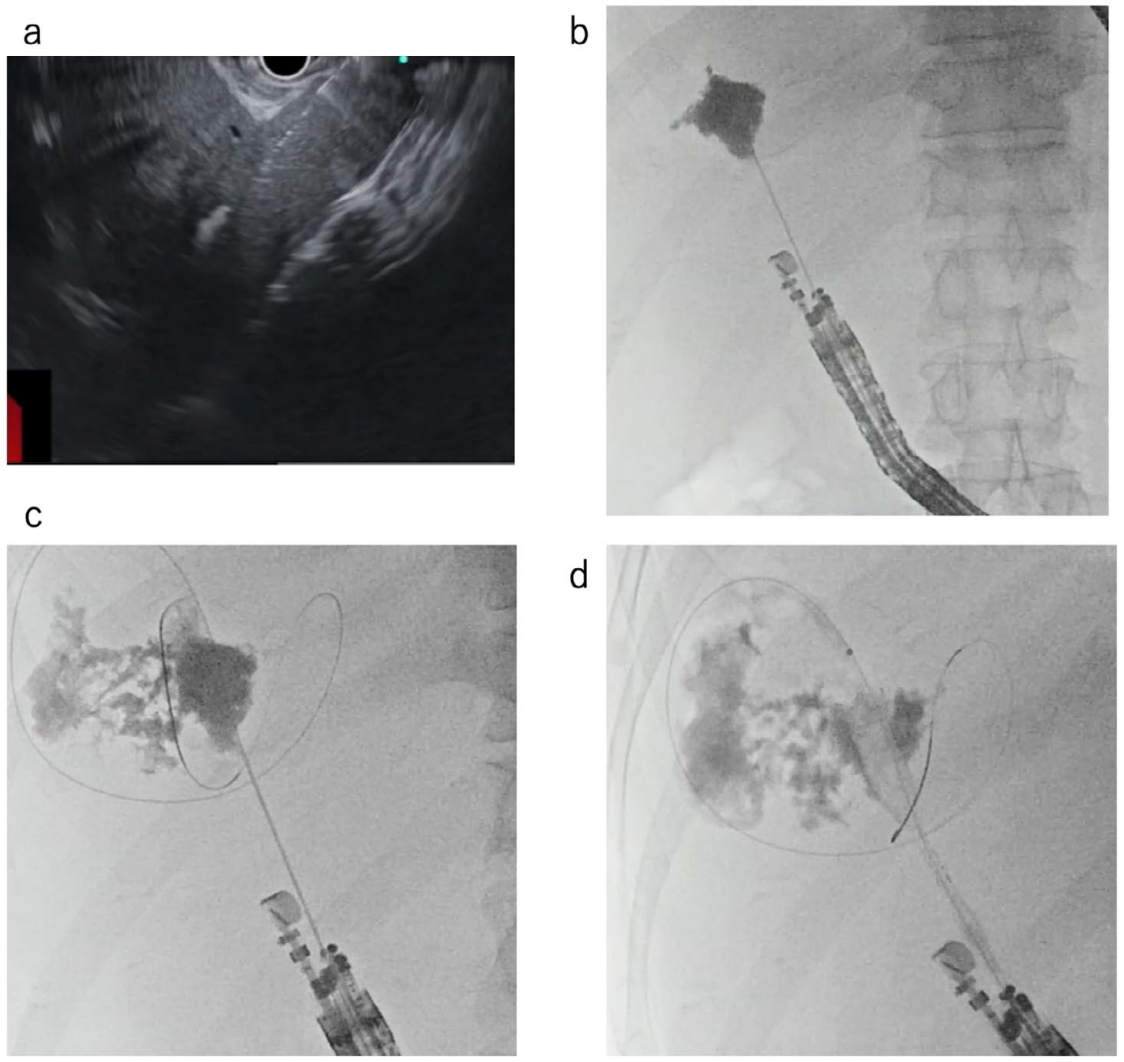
Technical tips for endoscopic ultrasound‐guided liver abscess drainage. (a) The liver abscess is punctured using a 19‐G needle. (b) The contrast medium is injected into the liver abscess. (c) A 0.025‐inch guidewire is deployed within the liver abscess. (d) Partially covered self‐expandable metal stent is placed from the liver abscess to the stomach or duodenum.
